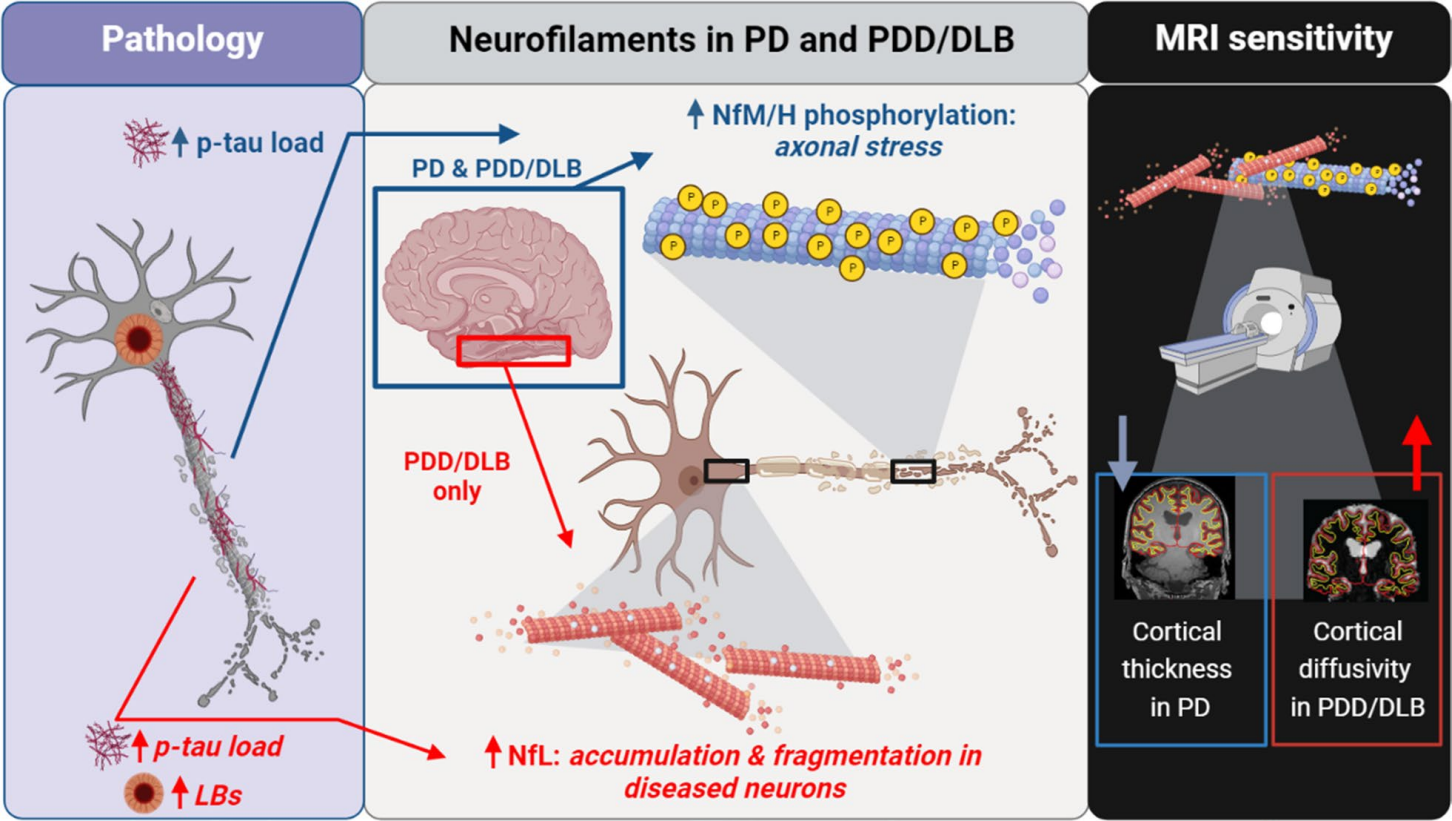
Increased neurofilament immunoreactivity is associated with pathology load and MRI biomarkers of neurodegeneration in PD. Middle panel: neurofilament immunoreactivity is increased in both PD and PDD/DLB cortex. Specifically, increased NfM/H phosphorylation occurs across the cortex in both PD and PDD/DLB (blue square), possibly indicating axonal stress, and is associated with increased p-tau load (left panel, blue arrow). On the other hand, an increased NfL immunoreactivity, representing NfL accumulation in disease neurons and fragmented axons, is observed in the entorhinal cortex and parahippocampal gyrus of PDD/DLB donors (red square) and associated with LB count and p-tau load (left panel, red arrow), suggesting structural changes with increasing pathological burden, and confirming its important role in the context of cognitive decline. Right panel: both increased p-NfM/H (i.e. axonal stress) and NfL immunoreactivity (i.e. NfL accumulation and fragmentation) are reflected by cortical thinning in PD and increased cortical MD in PDD/DLB. Figure created with BioRender.com. DLB: Dementia with Lewy Bodies; LB: Lewy Body; NfL: neurofilament light chain; PD: Parkinson’s disease; PDD: Parkinson’s disease dementia; NfM/H: phosphorylated neurofilament medium and heavy chain; p-tau: phosphorylated tau
